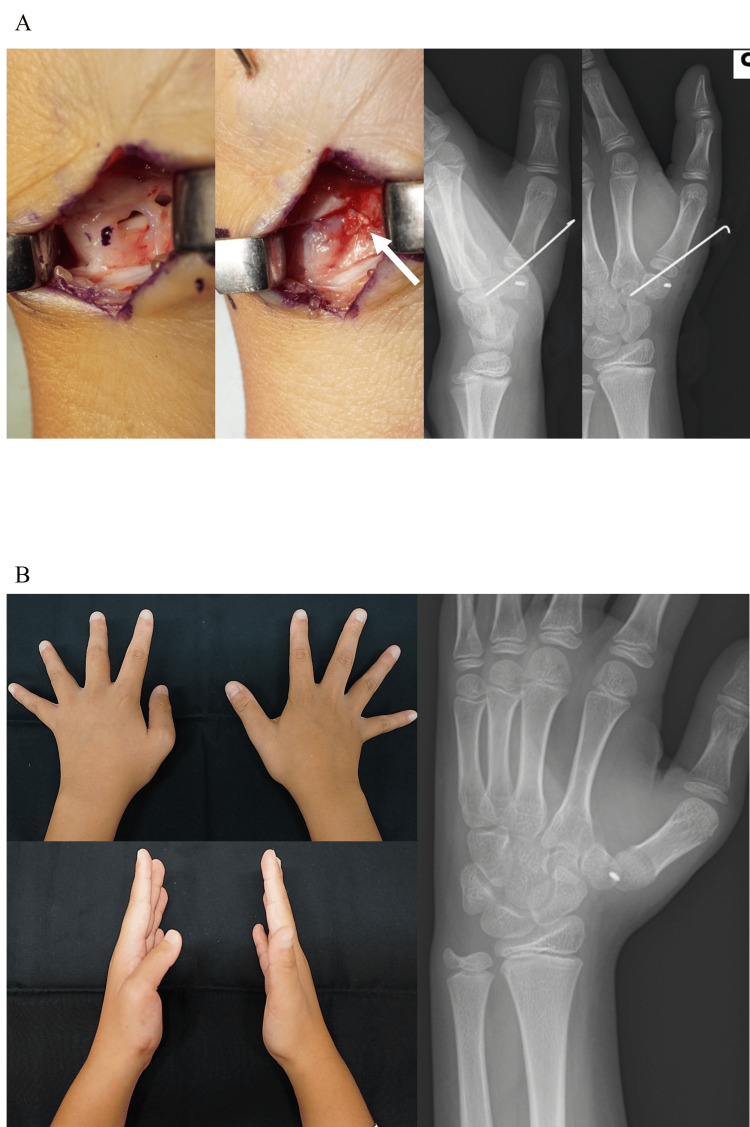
Images at and after the initial surgery. (A) Intraoperative finding of direct primary repair of the ligaments in the left thumb carpometacarpal (CMC) joint. The white arrow shows the sutured volar anterior oblique ligament. Postoperative radiograph showing the reduced CMC joint of the left thumb with percutaneous K-wire fixation.
